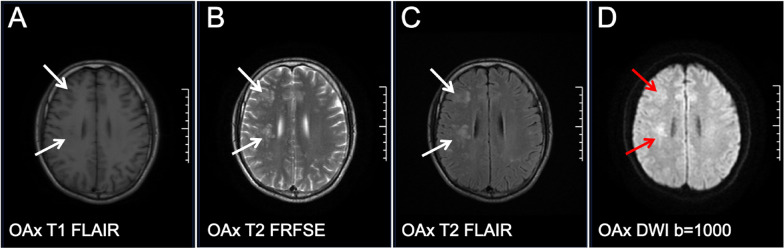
MR Plain scan results before admission (April 6, 2024). Craniocerebral MRI showed multiple nodular, lamellar and slightly longer T1 and slightly longer T2 signals in bilateral frontoparietal temporo-occipital lobe, right insula lobe, bilateral cerebellar hemispheres, and pontine, with borderless clearness, FLAIR with slightly higher signal, DWI with slightly higher signal, and ADC with slightly lower signal. A to D are MRI images at the level of OAx T1 FLAIR, OAx T2 FRFSE, OAx T2 FLAIR, and OAx DWI b=1000
